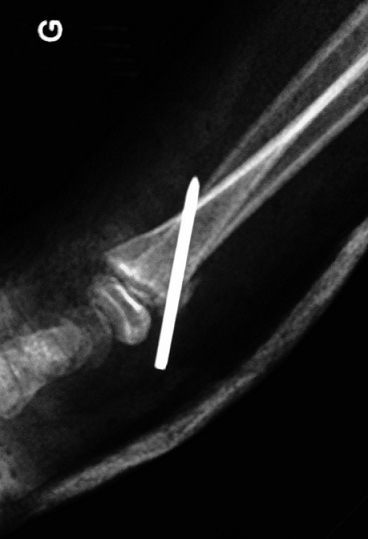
Radiograph of the wrist after reduction and pinning
